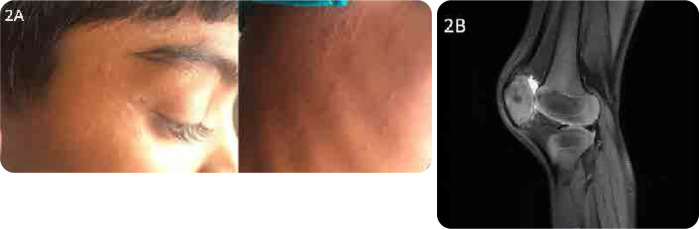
Patient 2. A. Shows urticarial erythematous papular rash with wheal upon presentation. B. MRI of the knee: enlarged patellae with irregular outlines suggestive of persistent NLRP3-associated arthropathy/hyperostosis.
